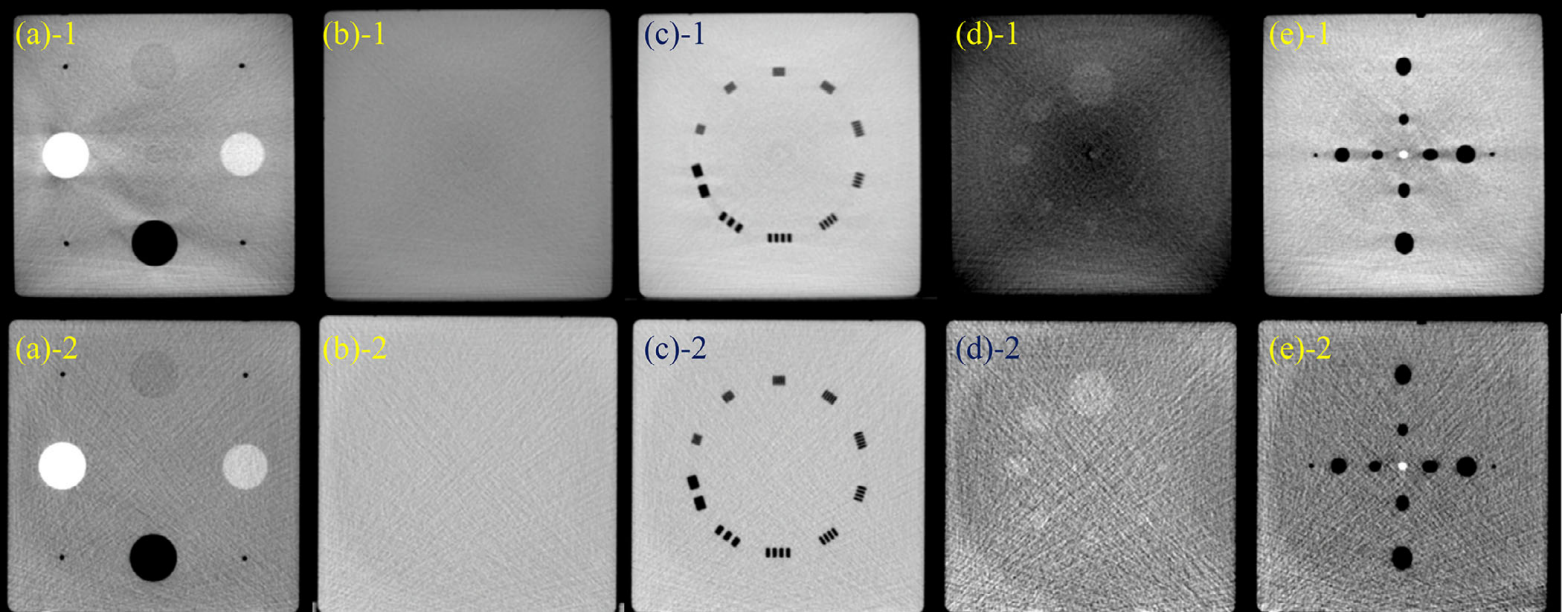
Transverse CBCT images from (1) XVI and (2) OBI: (a) HU constancy, (b) uniformity, (c) spatial resolution, (d) low contrast, (e) coincidence check.
